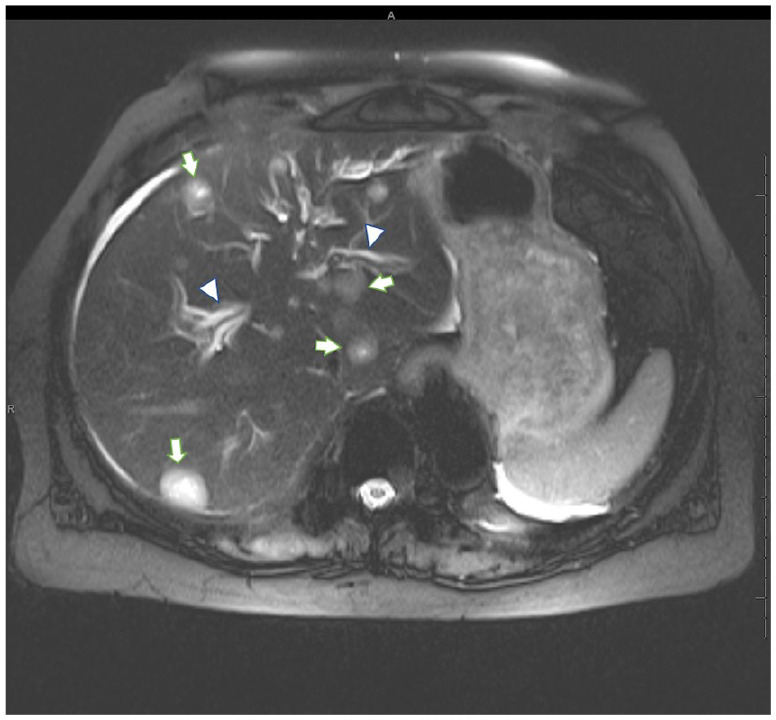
Axial T2 HASTE with fat saturation magnetic resonance image shows multiple intrahepatic T2 hyperintense lesions (white arrows), compatible with hepatic metastatic disease. Multiple dilated intrahepatic bile ducts (white arrowheads) noted as well.
